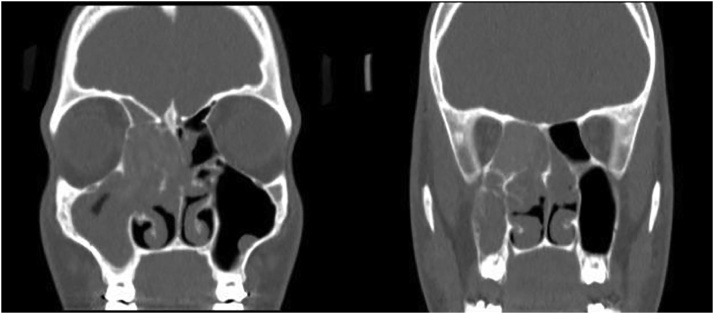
Preoperative imaging. In coronal view, the preoperative CT shows thick mucosal swelling outlining the antrum. Expanded ethmoid air cells are found on the right side. Radiopaque fungal elements are present within the opacified ethmoid air cells.
